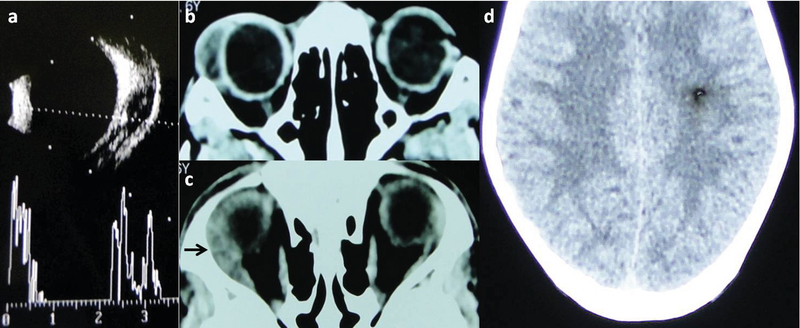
(a) B-scan ultrasound shows a hypoechoic lesion with high internal echogenicity suggestive of scolex in the right lateral rectus muscle. (b) Computed tomography axial scans show a cystic lesion abutting the right globe anteriorly. The heterogenous lesion has a hypodense cavity with a hyperdense internal spec suggestive of a scolex. (c) The superior axial section shows another heterogenous, posterior lesion in the belly of the lateral rectus muscle. (d) The left parietal lobe shows a hypodense lesion with a spec of internal hyper density, even though the classic ring-enhancement is absent.
